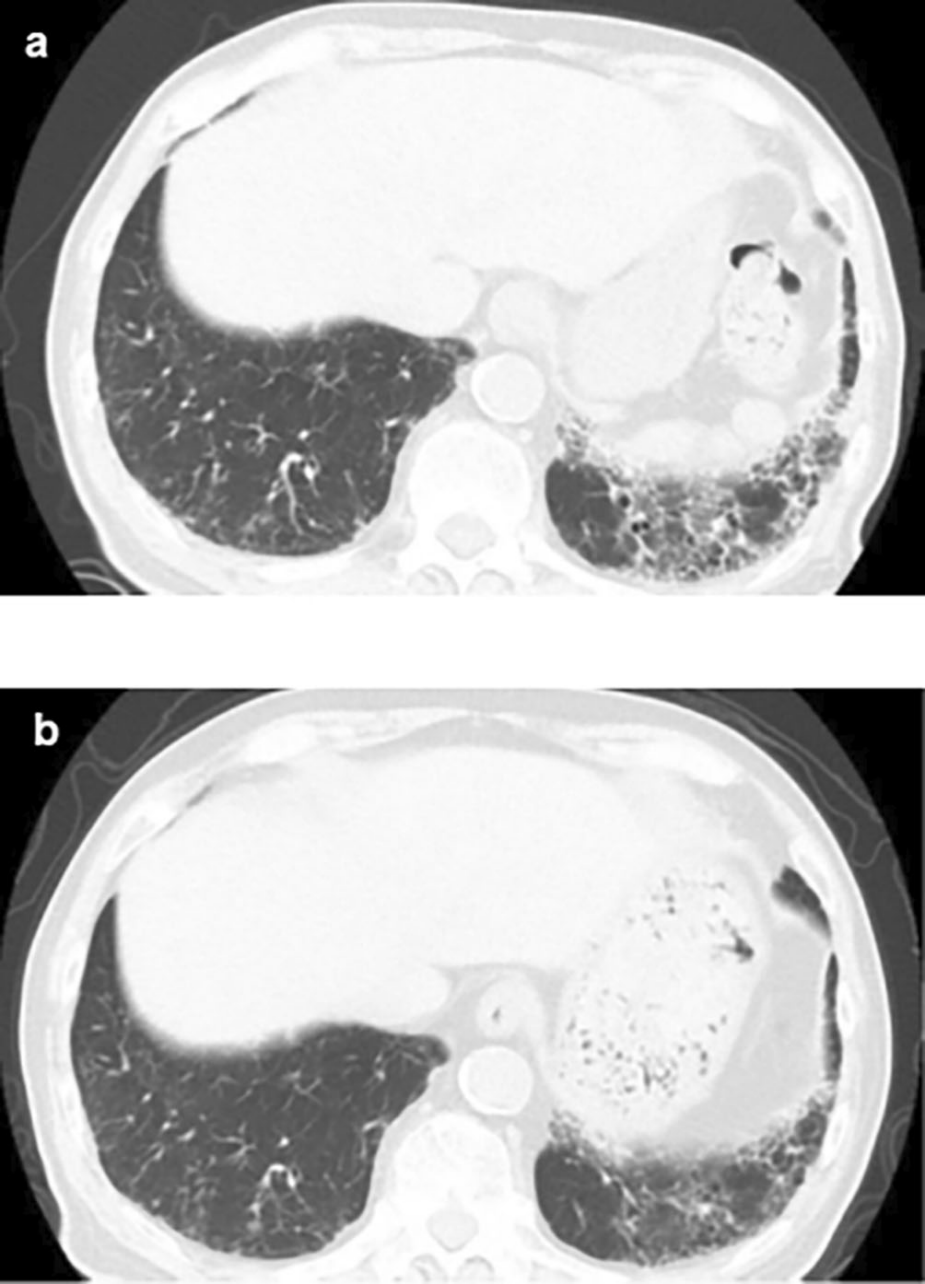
Computed tomography findings. (a) Plain chest CT showing the reticular pattern and bronchial dilatation in the bilateral lower lung fields before therapy; (b) After therapy, the interstitial pattern nearly disappeared from the bilateral lung fields. CT: computed tomography.
